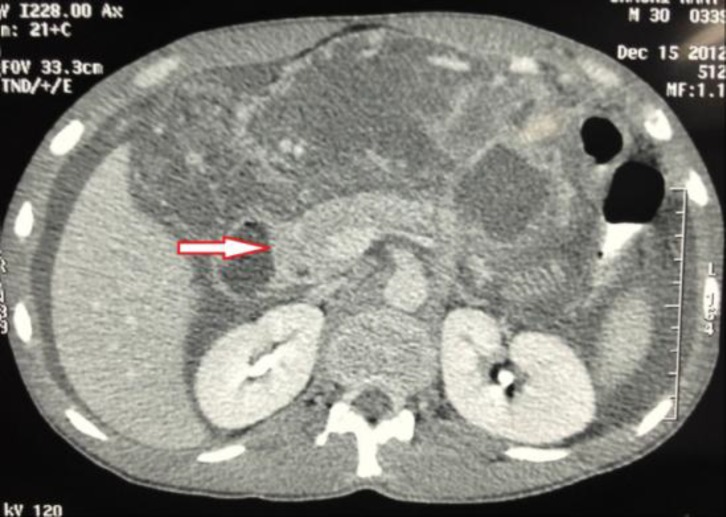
Contrast enhanced CT imaging of abdomen showing mild edematous pancreas.
